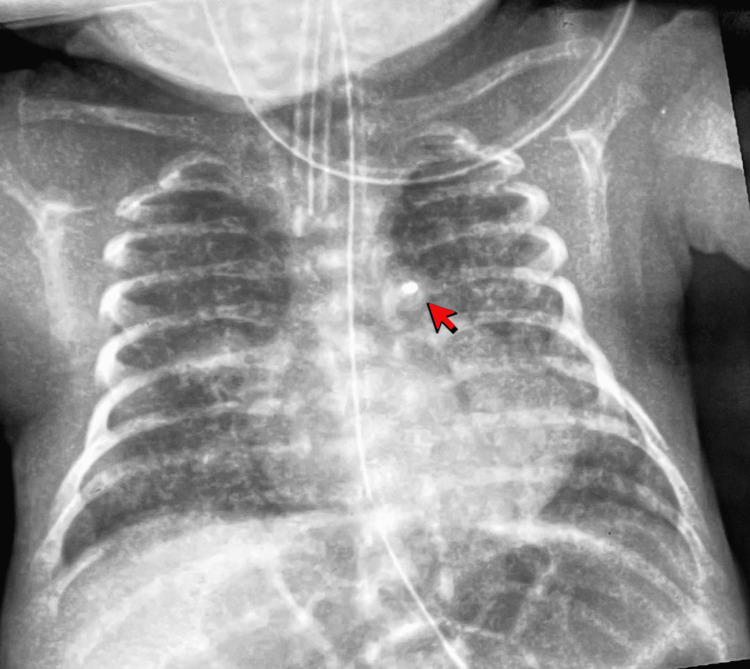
Post-procedural chest radiograph demonstrates the device in situ (red arrow). Note the interval reduction in cardiothoracic ratio and reduced pulmonary vascular marking compared to the pre-procedure chest x-ray.
